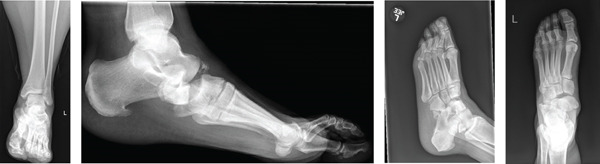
Anteroposterior and lateral injury radiographs.
